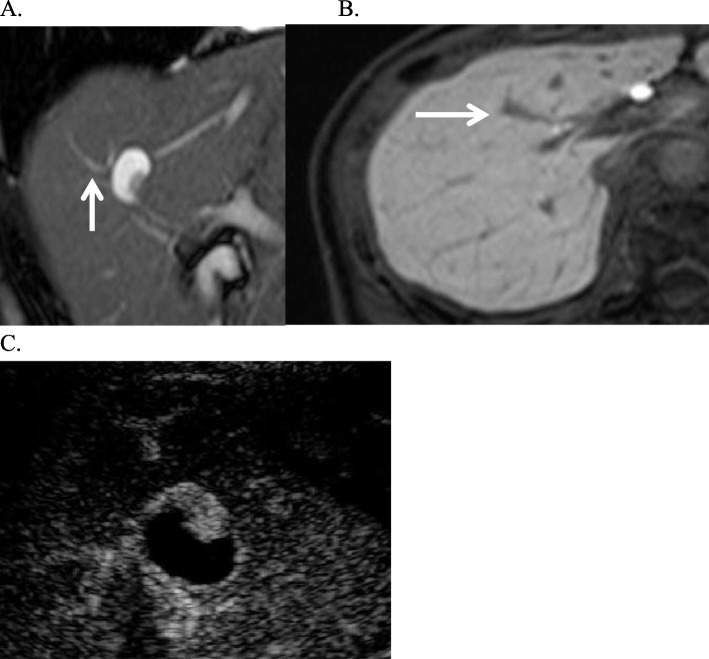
a, b Magnetic resonance imaging findings. c Ultrasonography findings. Magnetic resonance imaging showed slight dilation of the distal bile duct (white arrow). Ultrasound showed a highly echoic papillary nodular area in the cystic lesion
